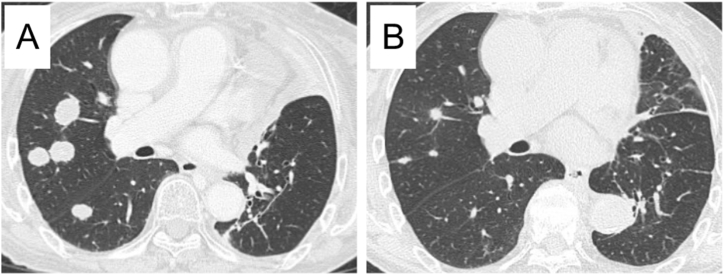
Chest computed tomography findings. Images before the administration of osimertinib showed enlarged metastatic colonies predominantly in the right lung (A). After two months of osimertinib treatment, the metastatic colonies had obviously shrunk (B).
